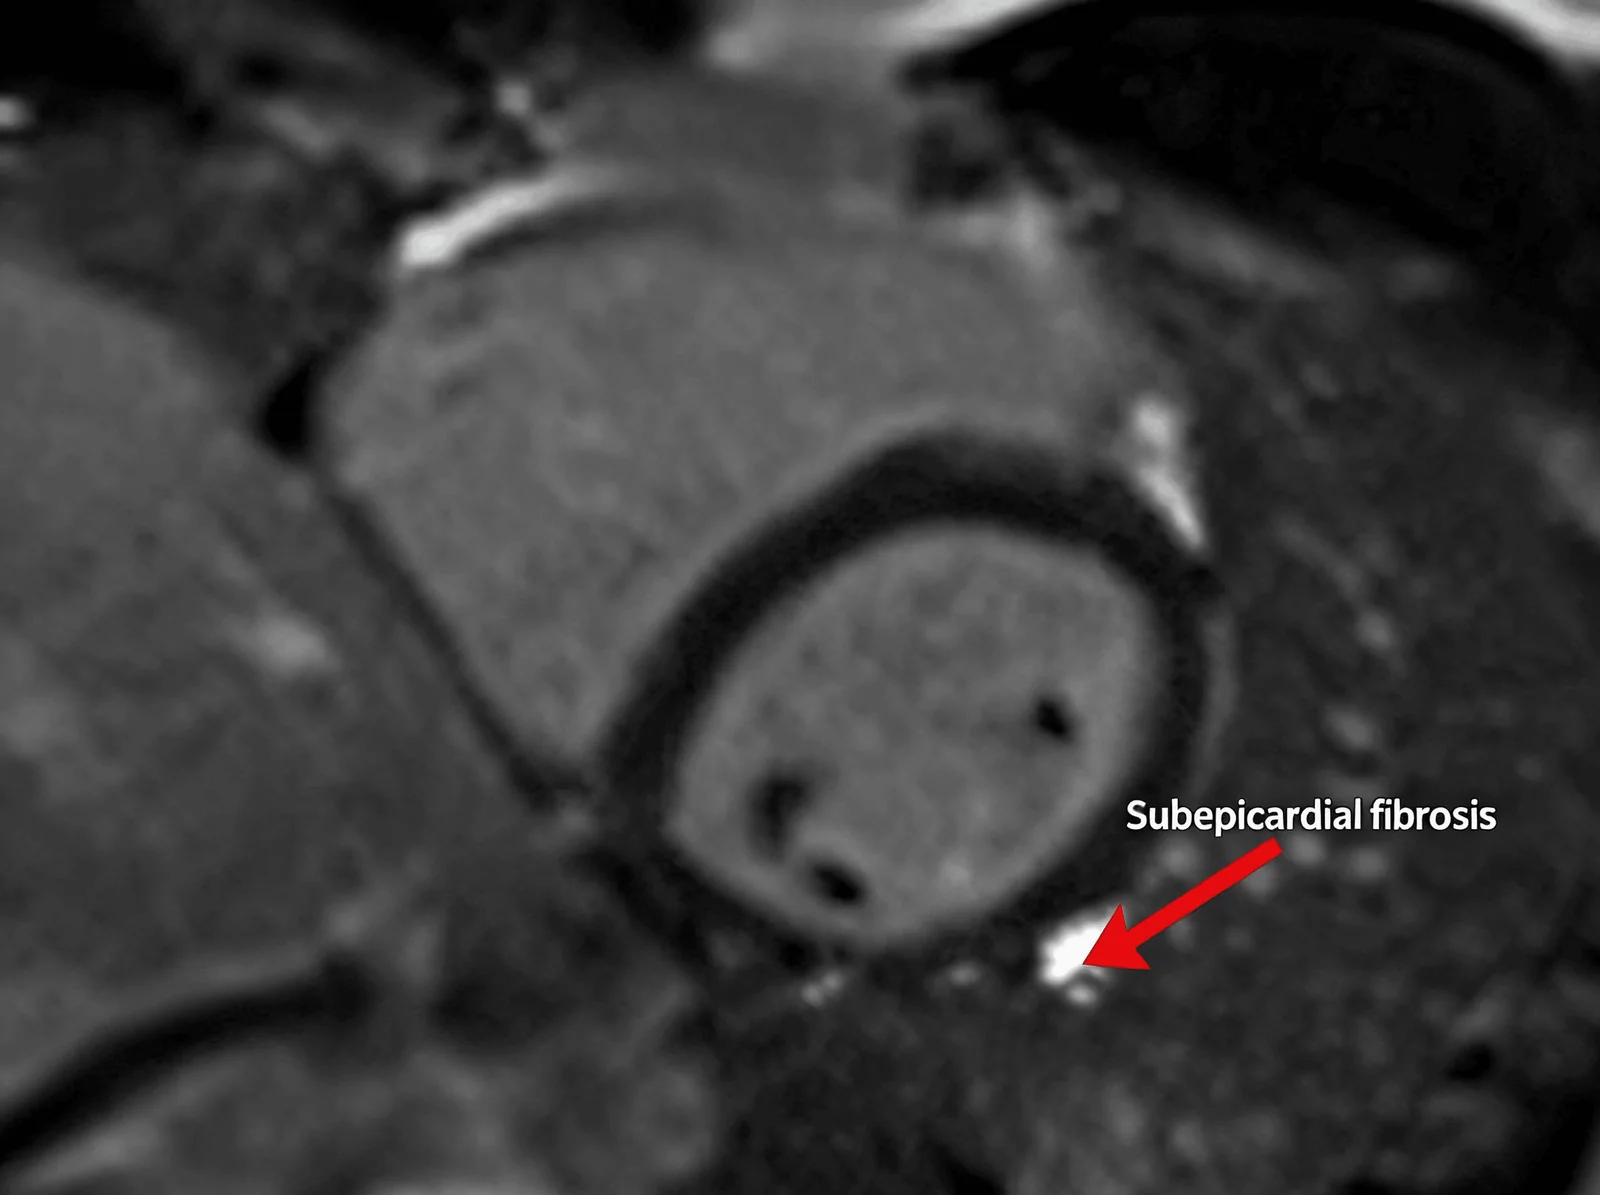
Cardiac magnetic resonance imaging demonstrating subepicardial fibrosis of the basal inferolateral wall. Late gadolinium enhancement cardiac magnetic resonance imaging demonstrating focal subepicardial fibrosis in the basal inferolateral left ventricular wall (red arrow). The non-ischaemic enhancement pattern is consistent with prior myocarditis and may represent a substrate for ventricular arrhythmogenesis.
